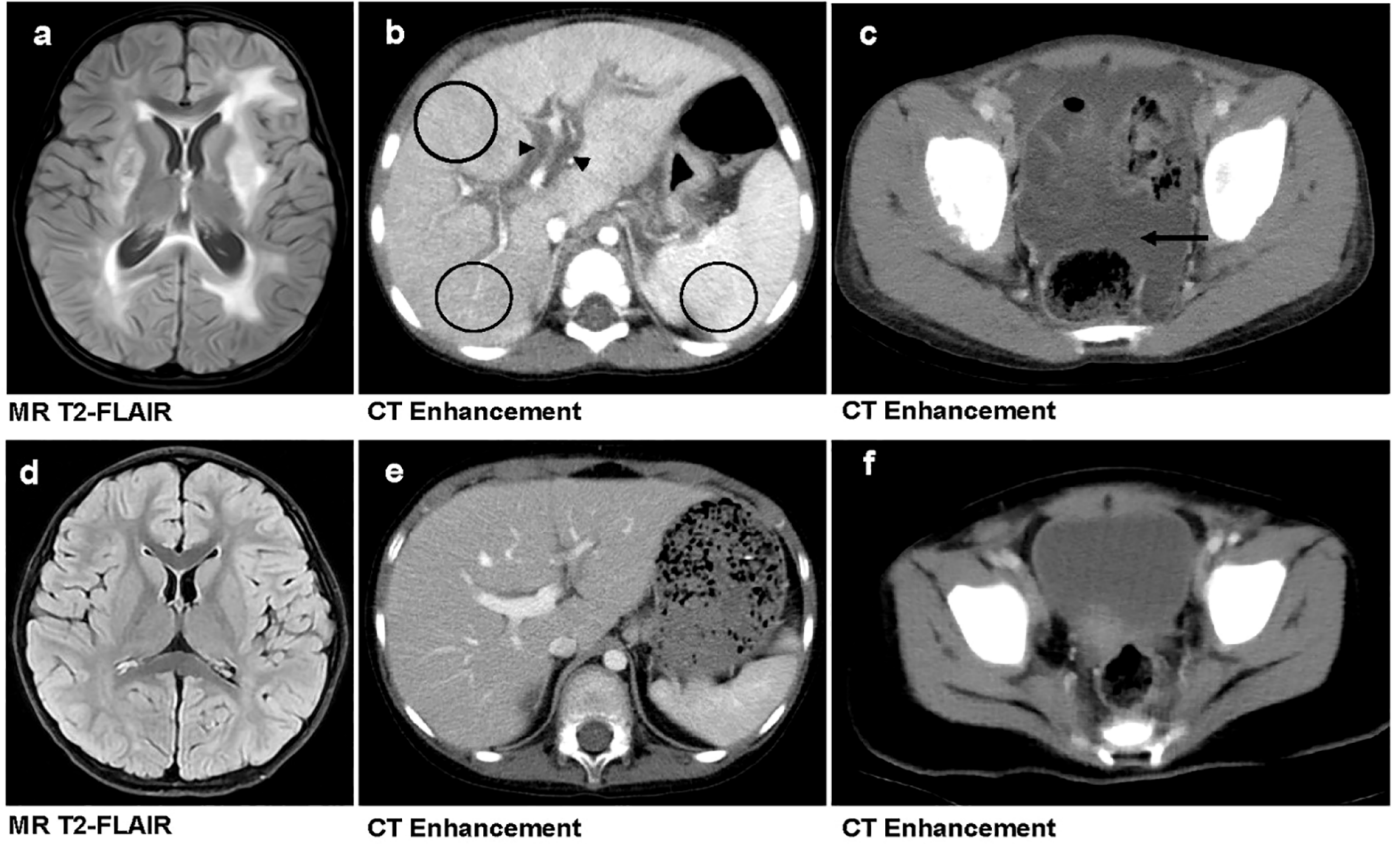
Comparison of imaging findings between primary and secondary HLH in pediatric patients. (a–c) A 3-year-old girl with primary HLH (genetic defect: UNC13D, familial HLH type 3 [FHL3]) who succumbed to the disease. Brain MRI reveals widespread bilateral T2-FLAIR hyperintensities, indicative of parenchymal involvement (a). Contrast-enhanced abdominal CT demonstrates hepatic interstitial edema (marked by black triangles) and heterogeneous hepatosplenic enhancement (highlighted by black circles) (b). Contrast-enhanced pelvic CT shows massive ascites (indicated by black arrow) (c). (d–f) A 3-year-old boy with secondary HLH who survived. Brain MRI (d), contrast-enhanced abdominal CT (e), and Contrast-enhanced pelvic CT (f)—all show no significant abnormalities.
